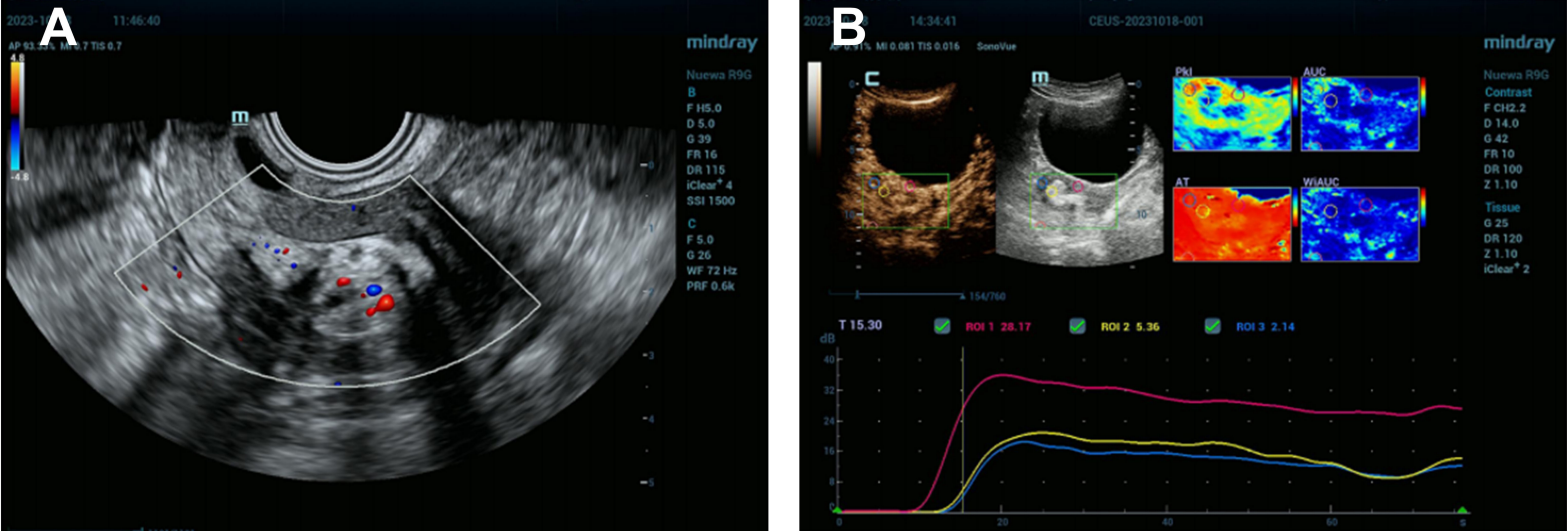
Uterine Mixed Echogenic Lesion Revealed by Comprehensive Ultrasonography and Enhanced Imaging Studies. (A) Preoperative transvaginal ultrasound images showed a normal uterine morphology with a uniformly thin endometrium. A mixed-echo lesion was observed within the uterine cavity, measuring 30 mm × 17 mm × 21 mm. The images suggested a submucosal leiomyoma with metaplasia or an endometrial polyp. No obvious masses were detected in the bilateral adnexa. The left ovary measured 12 mm × 7 mm, and the right ovary measured 12 mm × 6 mm. (B) Enhanced tumor image displaying late high enhancement relative to the muscle layer, followed by late low enhancement, indicative of submucous leiomyoma with metaplasia.
